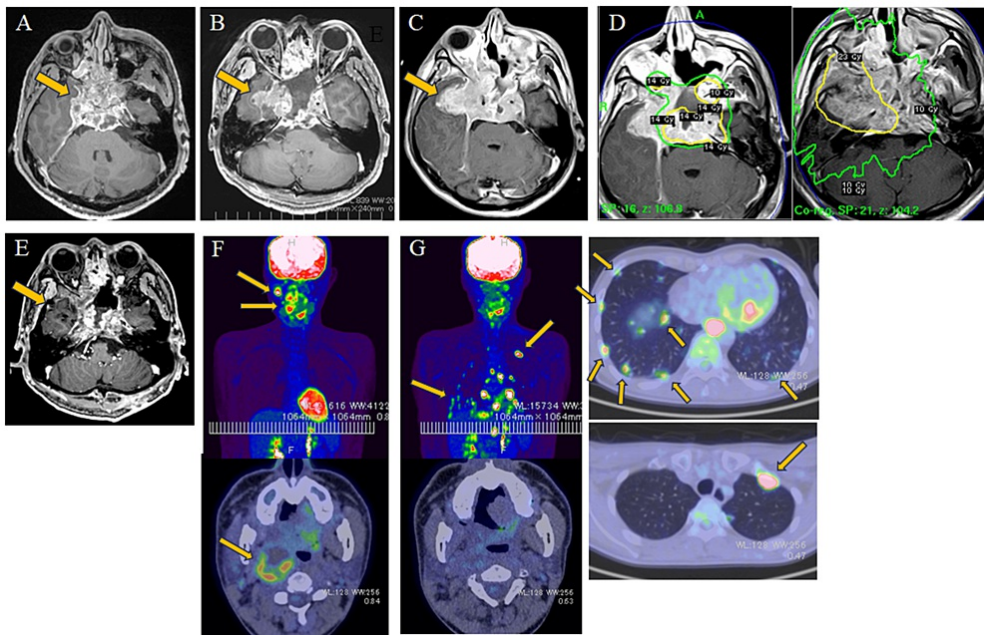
Images during the course of the disease. MRI showing preoperative findings (A), third day after surgery (B), and two weeks after surgery (C). A 14-Gy dose was delivered to the tumor margin at the 40% isodose (yellow line) for a lesion from the cavernous sinus to the left middle cranial fossa (D left panel) and a 23-Gy dose was delivered to the tumor margin at the 40% isodose (yellow line) for a lesion from the right side of the clivus to the right middle cranial fossa (D right panel). Two months after irradiation by a gamma knife (E). Fludeoxyglucose-18 positron emission tomography three months after surgery (F) and 5.5 months after surgery (G).
